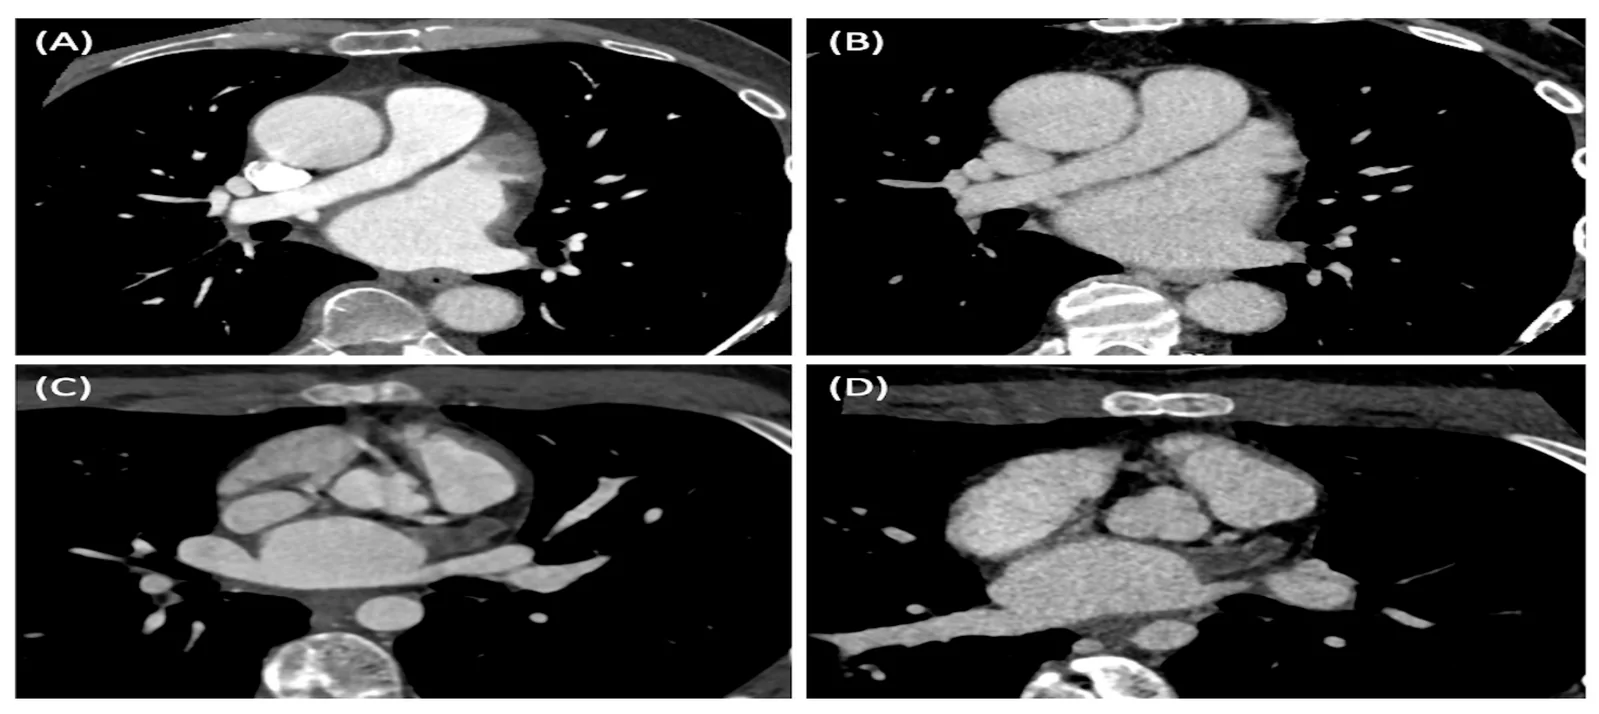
Thrombus rule-out with CT: angiographic phase showing a suspected filling defect of the LAA (panel (A)) and delayed phase of the same patient demonstrating normal opacification of the left appendage (panel (B)); other angiographic-phase image showing a suspected LAA thrombus (panel (C)) with corresponding delayed-phase image confirming the presence of thrombus within the LAA (panel (D)).
